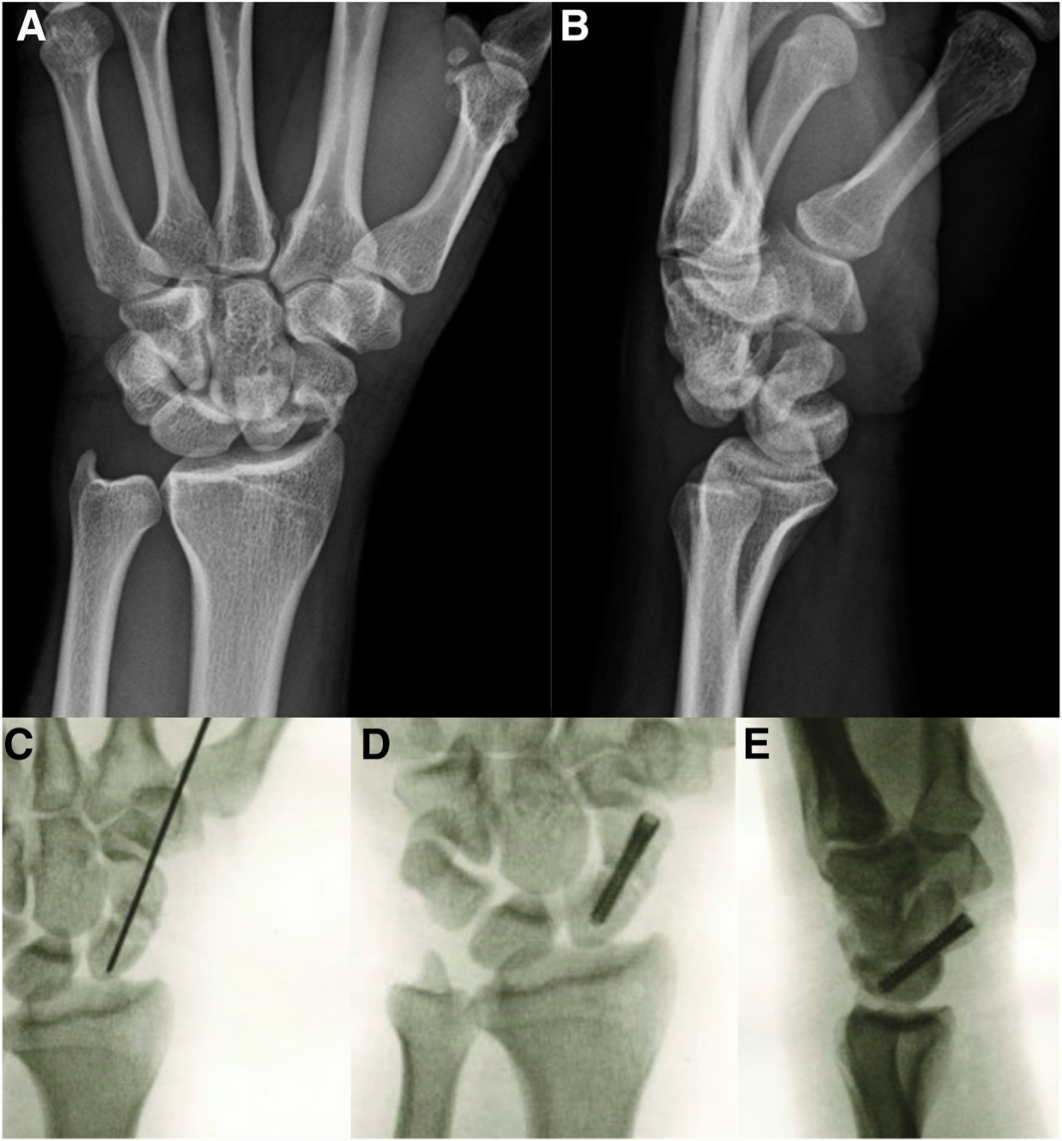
Prereduction radiographs of a left transscaphoid perilunate injury (A and B) sustained by a 24-year-old male while playing basketball. After closed reduction is performed, the patient is taken to the operating room for arthroscopic assisted management of the APLI. The scaphoid fracture is addressed initially. This was performed via a percutaneous volar technique. Intraoperative fluoroscopy demonstrates reduction and stabilization of the scaphoid with a Kirschner wire (C), followed by the insertion of a headless compression screw, with anatomic reduction of the scaphoid (D and E).
